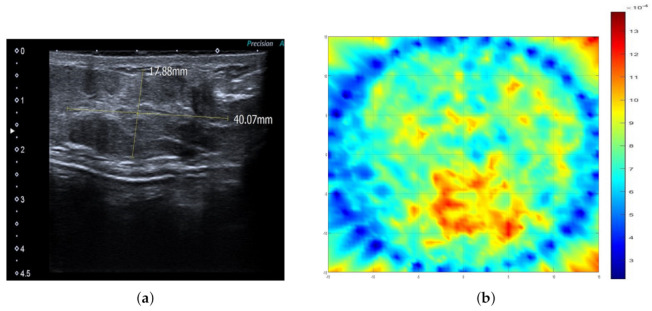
Comparison of SAFE image with clinical ultrasound: (a) ultrasound scan showing benign lesion in the left breast and (b) SAFE scan showing no concordance with ultrasonography because of air gaps.
